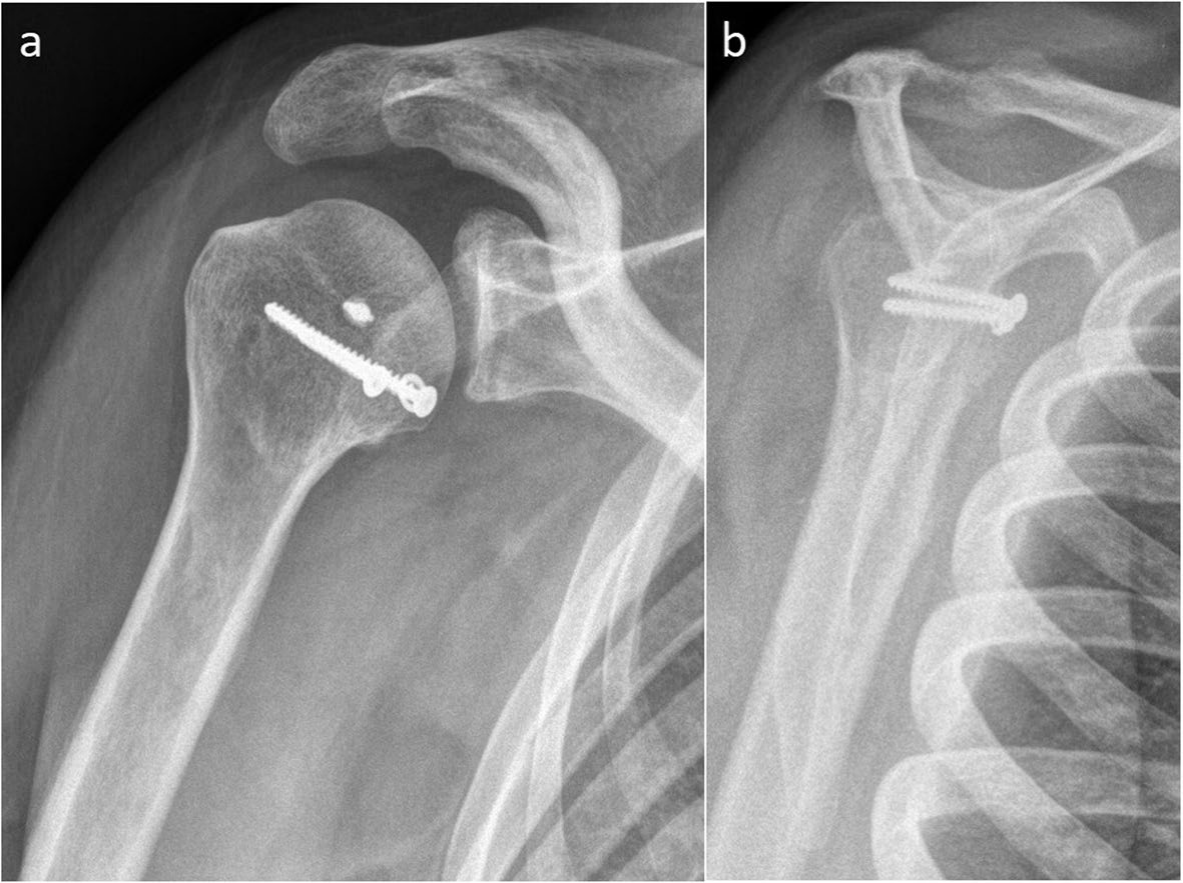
Locked posterior shoulder dislocation (right shoulder), postoperative imaging. a Anteroposterior and (b) scapular Y view X-ray images of the right shoulder of a patient with locked posterior shoulder dislocation treated with the modified McLaughlin procedure
